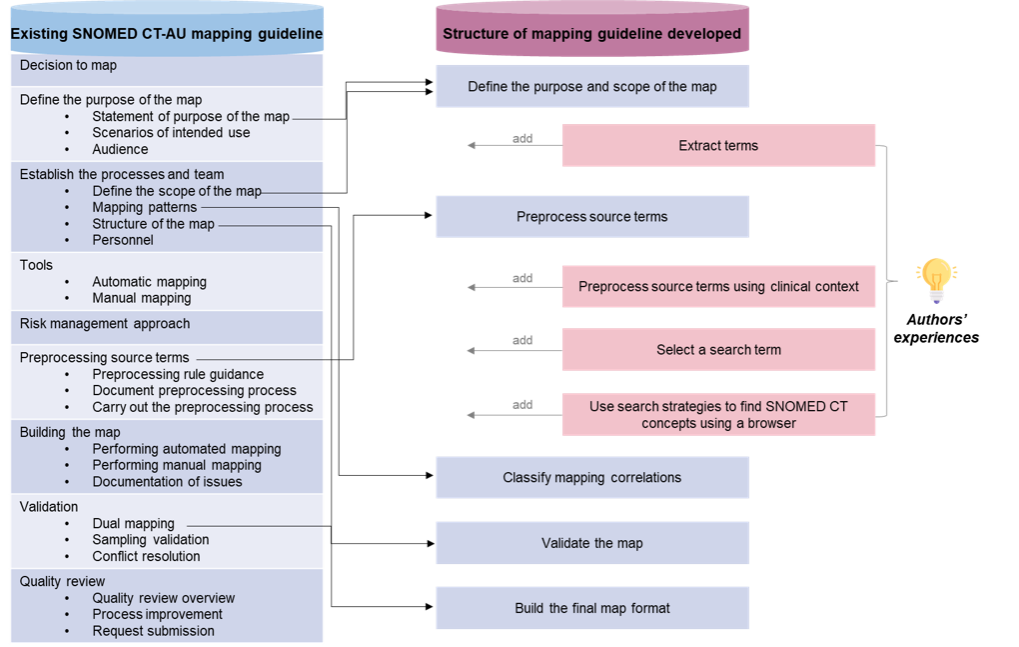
Overview of the developed guideline structure. SNOMED CT: Systemized Nomenclature of Medicine–Clinical Terms.
